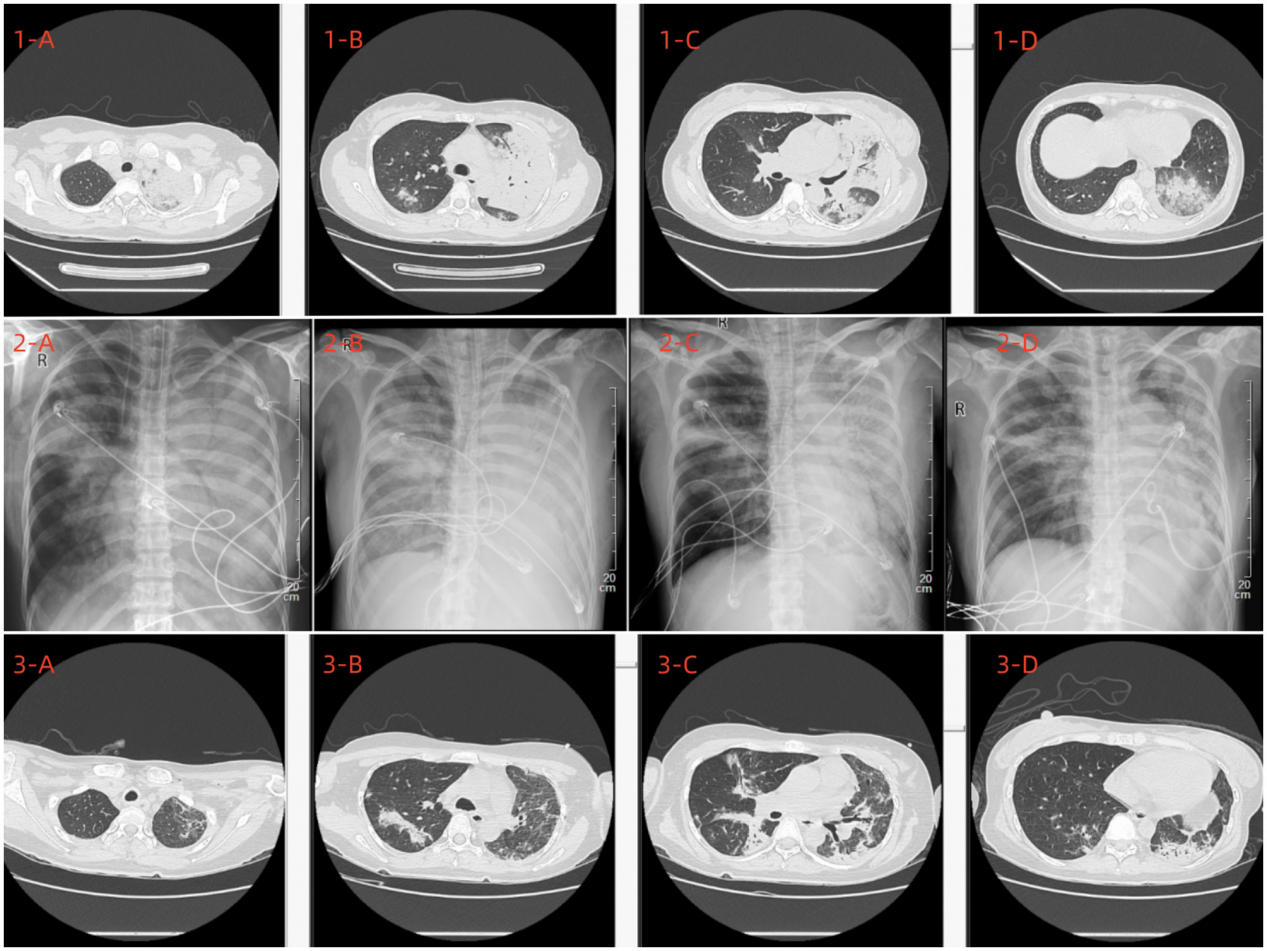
Upon admission, a chest CT scan (1A–D) revealed multiple exudative lesions throughout both lungs, predominantly in the left lung. (2A–D) Depict the chest X-ray findings on the 6th, 8th, 15th, and 18th days post-admission, respectively. A subsequent chest CT conducted on the 22nd day post-admission (3A–D) indicated a significant reduction in the size of the lesions in both lungs.
